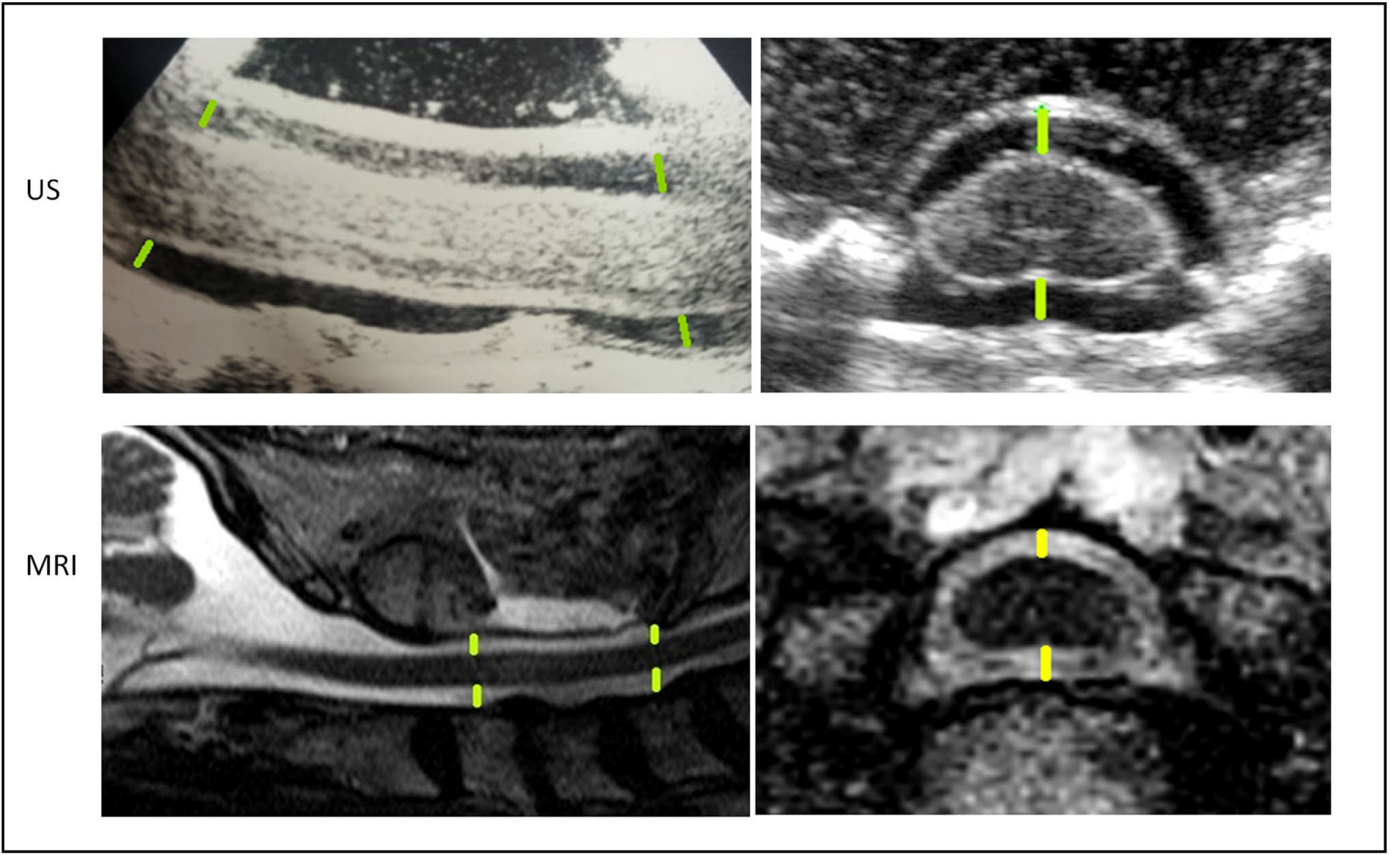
Upper line: ultrasonography of the spinal cord in sagittal and axial plane. Lower line: sagittal and axial postoperative MRI (T2-weightened). Locations of measurements are inserted in green
